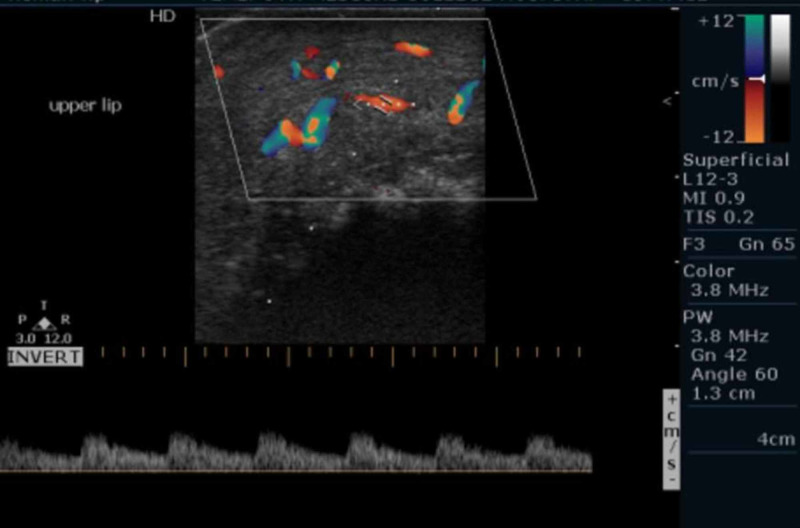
Color doppler application on USG showing moderate uptake by mixed blue and red color suggesting AVM.‎ USG, ultrasonography; AVM, arteriovenous ‎malformation
